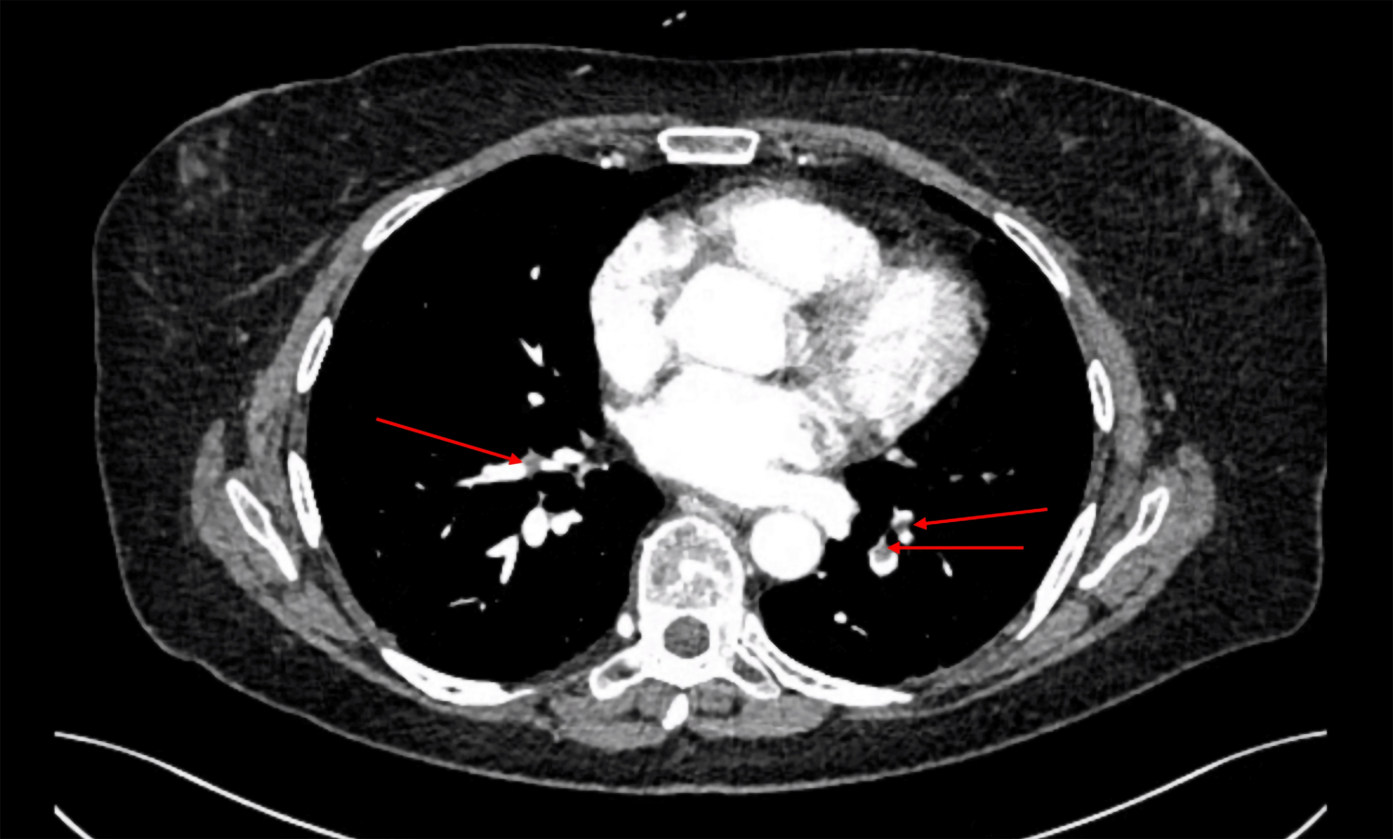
Axial image from CTPA that demonstrates bilateral segmental pulmonary emboli. CTPA: computed tomography pulmonary angiography
